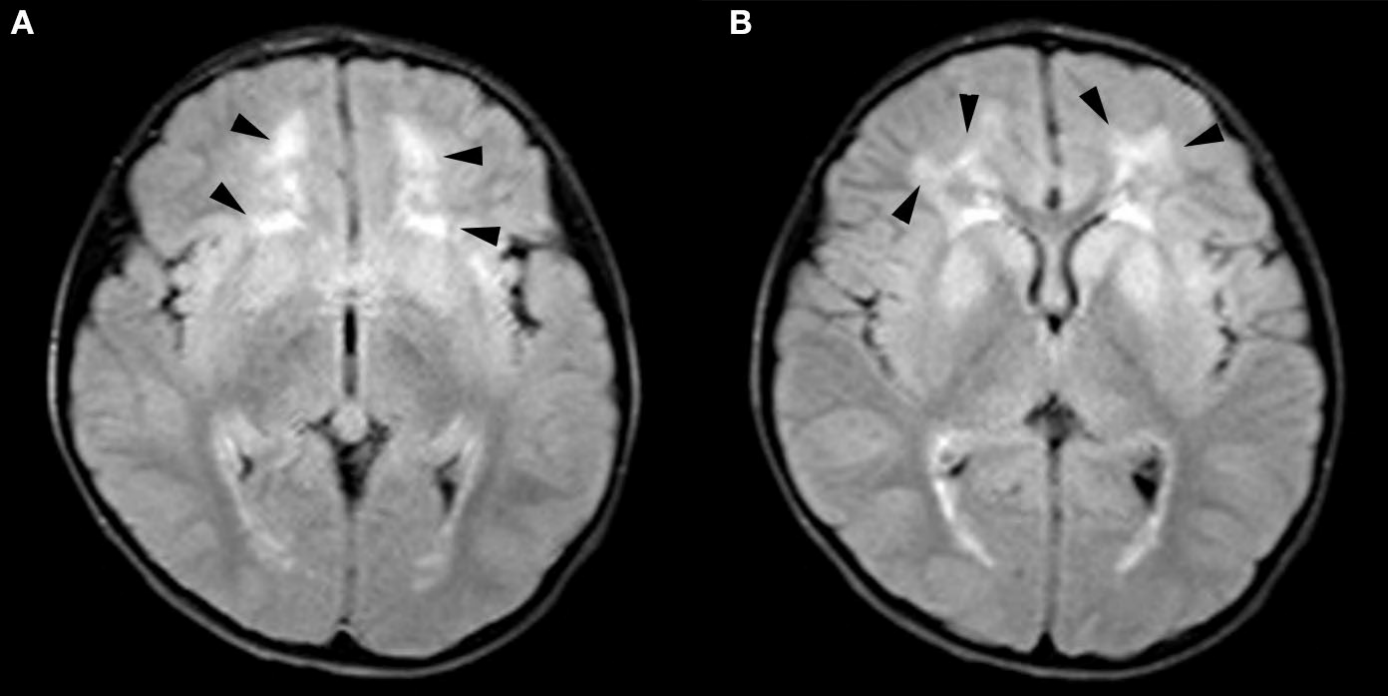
Brain magnetic resonance imaging (MRI): (A) Bilateral subfrontal cortex and (B) bilateral paraventricular abnormal signals, as displayed by the arrow.
